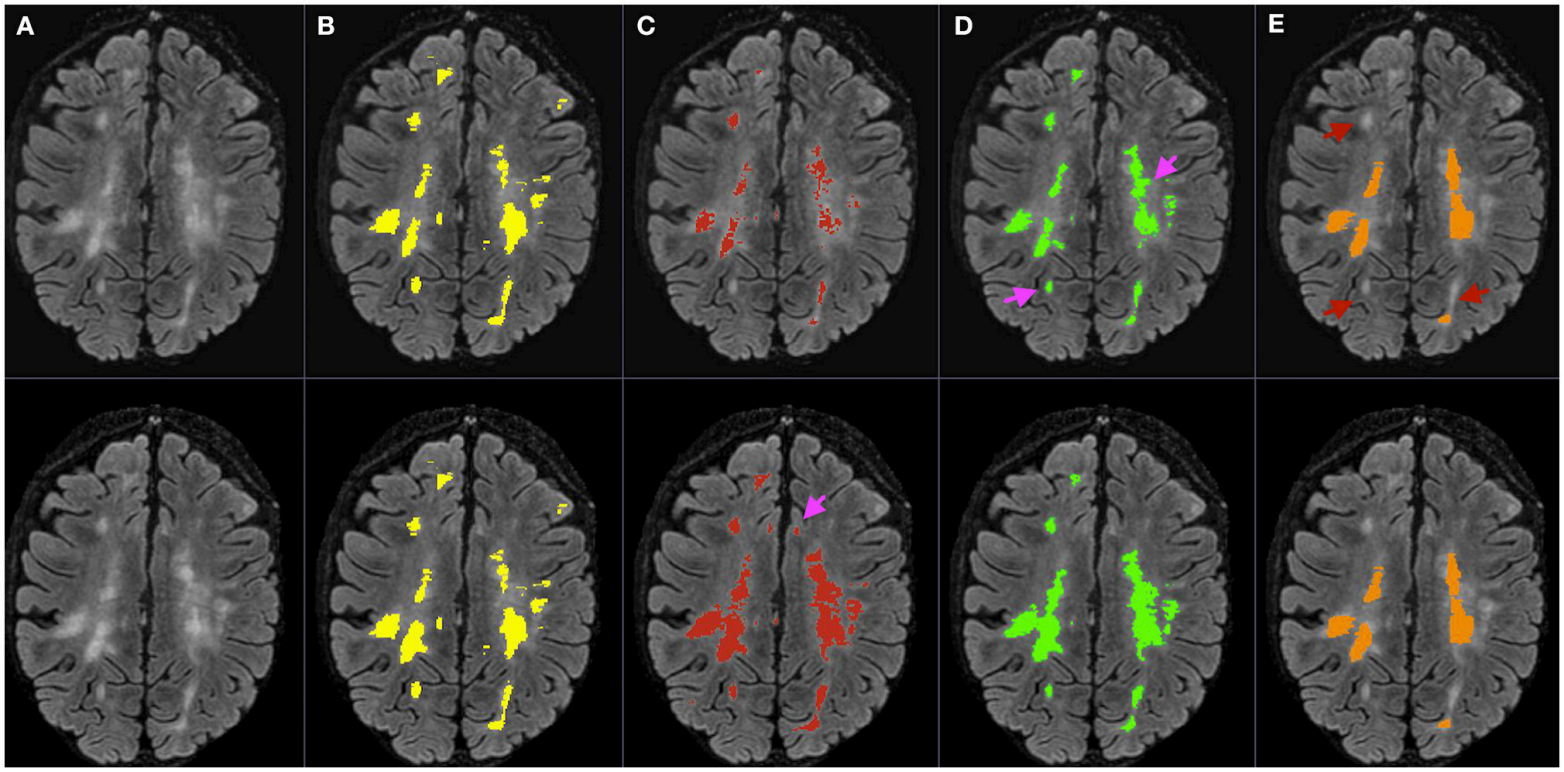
Bias corrected FLAIR image (A) followed by super-imposed lesion segmentation from: (B) expert segmentation, (C) MSmetrix-cross (version 1.4), (D) MSmetrix-long, and (E) LST-long. The first row corresponds to the lesion segmentation of time point 1 and the second row corresponds to the lesion segmentation of time point 2. Pink arrows specify places where MSmetrix-long has improved in accuracy over MSmetrix-cross and red arrows indicate regions where LST-long has missed lesions.
